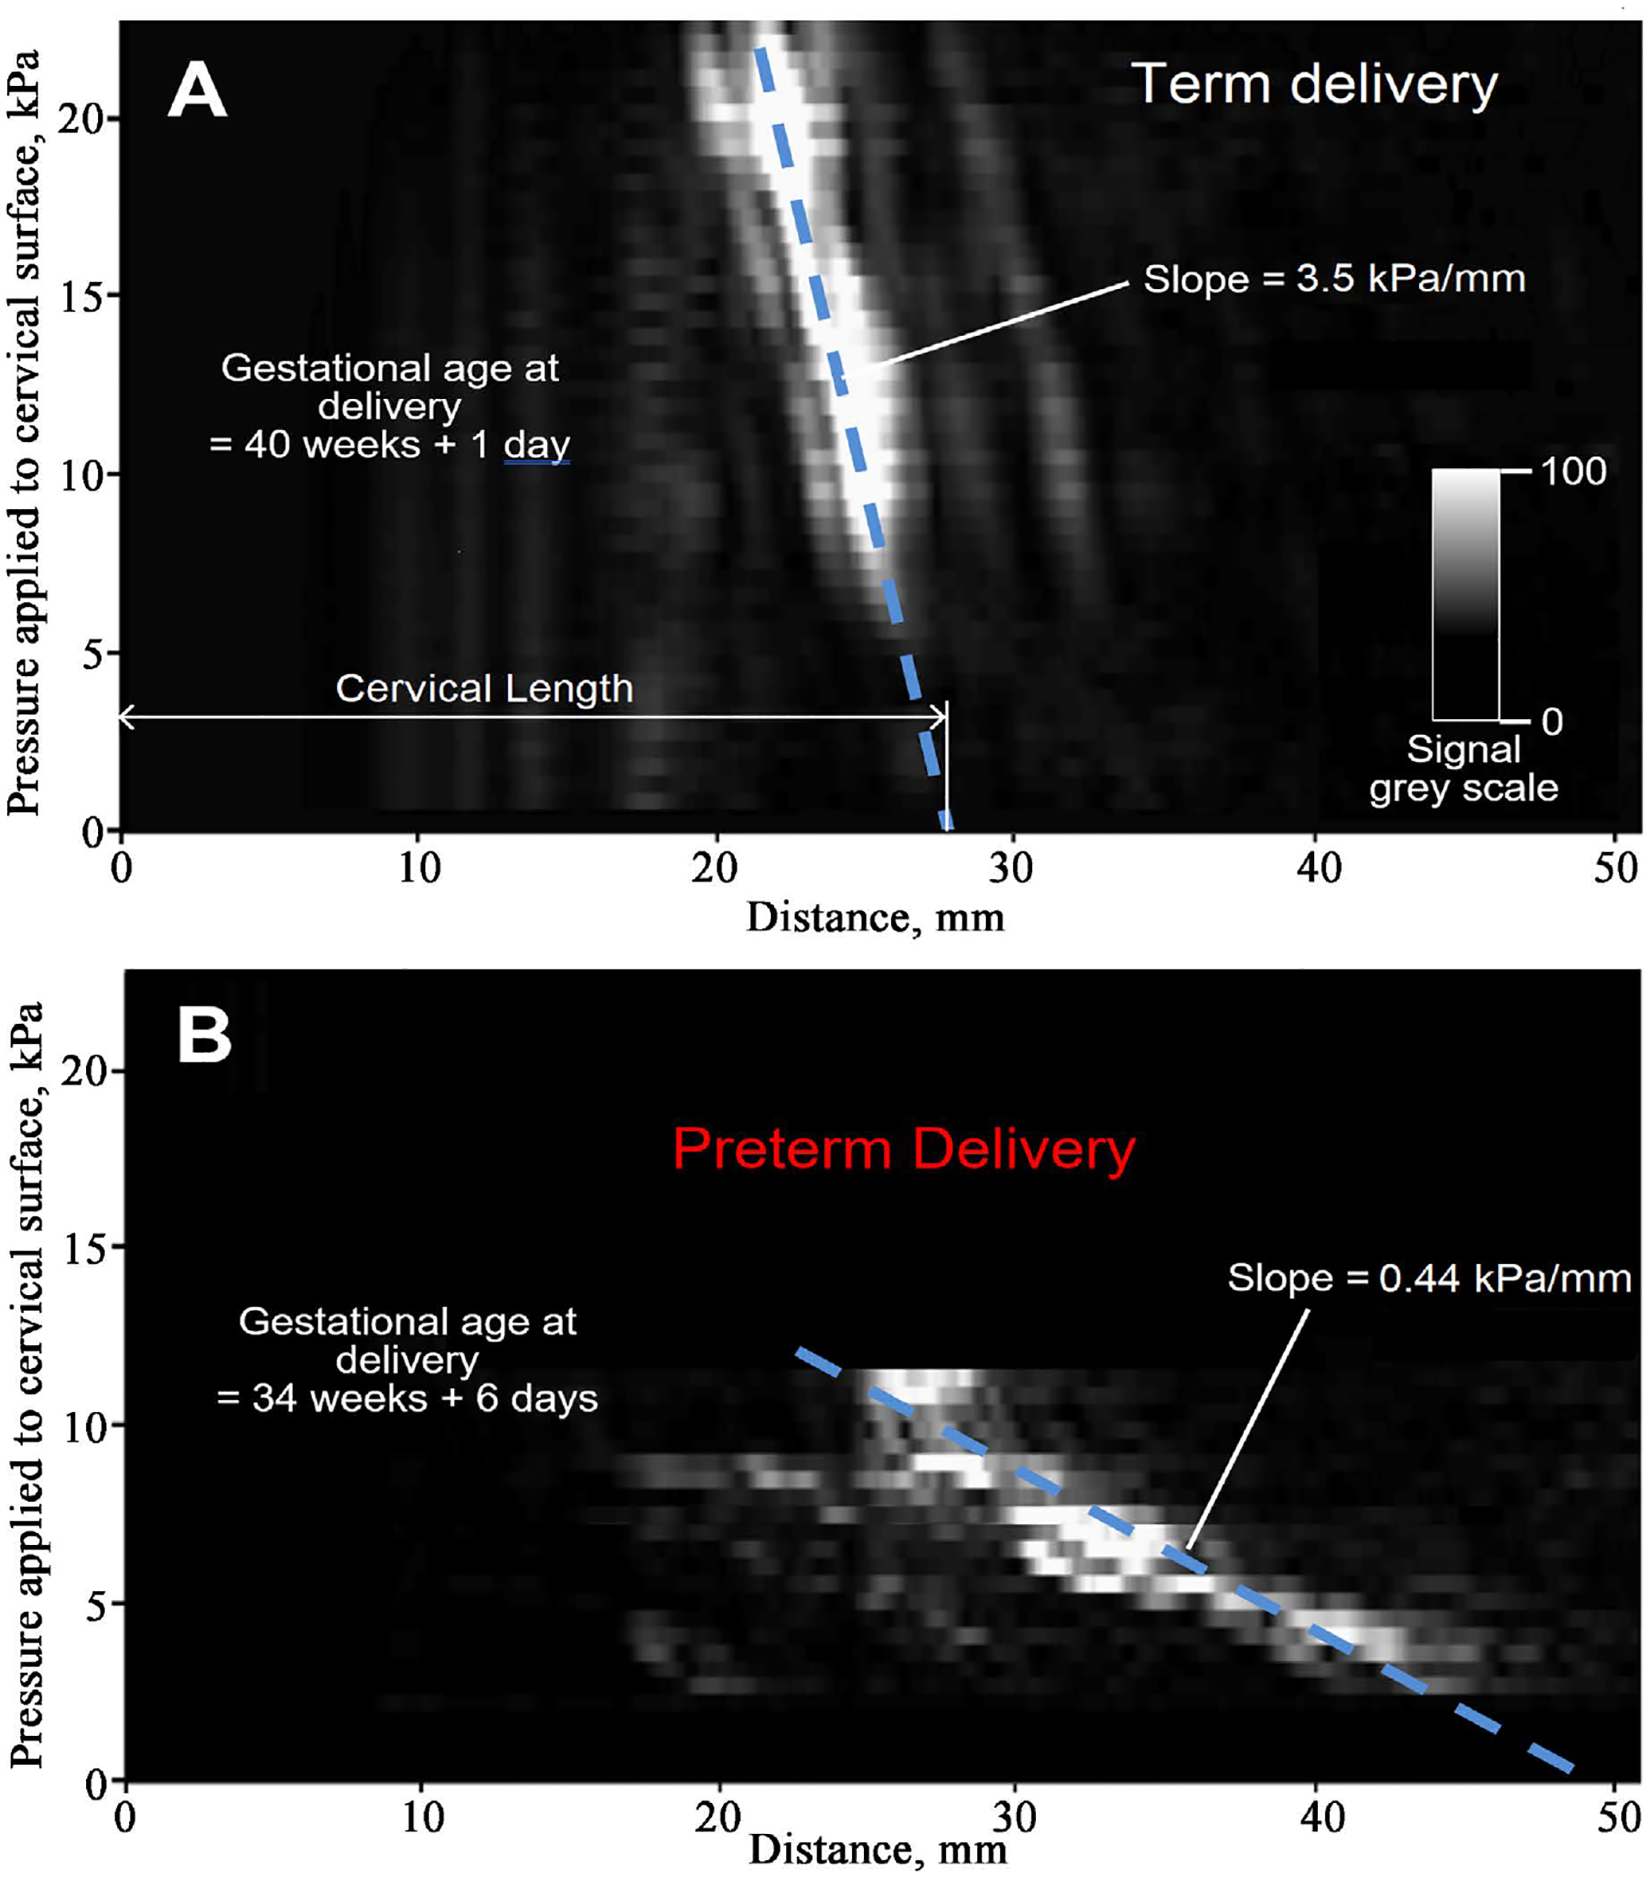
Stress-strain cervical mapping using ultrasound signals reflected from the internal cervical surface and applied pressure at cervix by the CM probe, acquired at 24 – 28 gestational weeks. (A) term birth at 40 and 1 day, (B) preterm birth at 34 weeks and 6 days.
